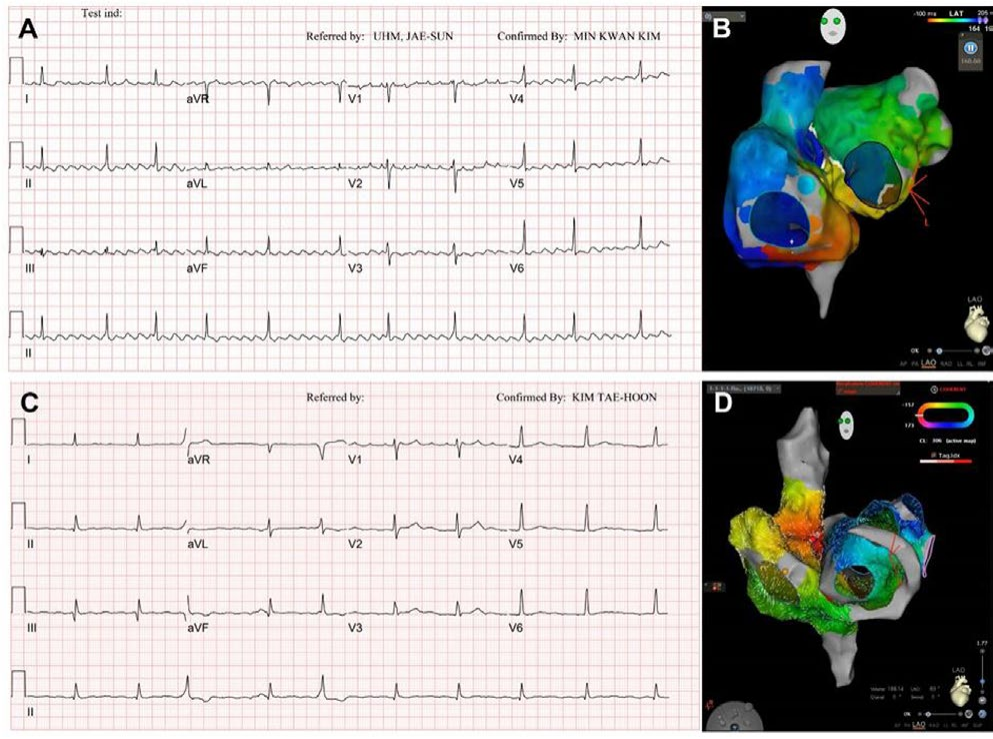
## EVALUATION OF THE ACTUAL IMPLANTATION SITES OF LEADLESS PACEMAKERS USING COMPUTED TOMOGRAPHY (CT) IMAGES
